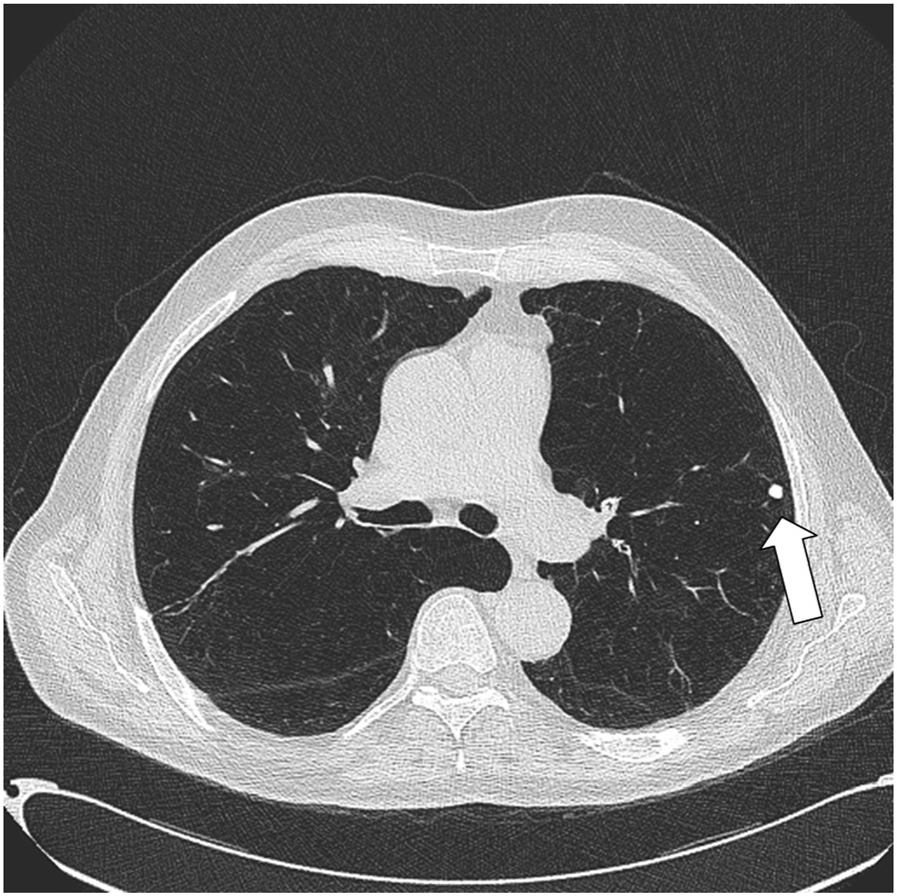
Chest CT scan showing emphysema and nodule (arrow).
